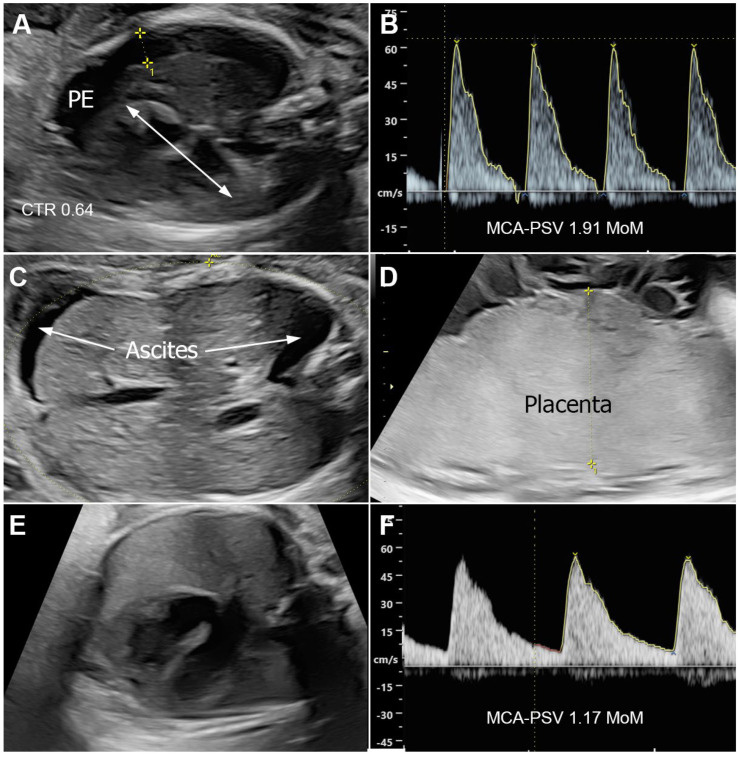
Ultrasound findings of Case 6; (A–D) (26 weeks) show hydrops; pleural effusion (PE) and cardiomegaly (A), high MCA–PSV (B), ascites (C), and placentomegaly (D); (E,F) (33 weeks) show disappearance of hydrops; no pleural effusion (E) and normal MCA–PSV (F).
